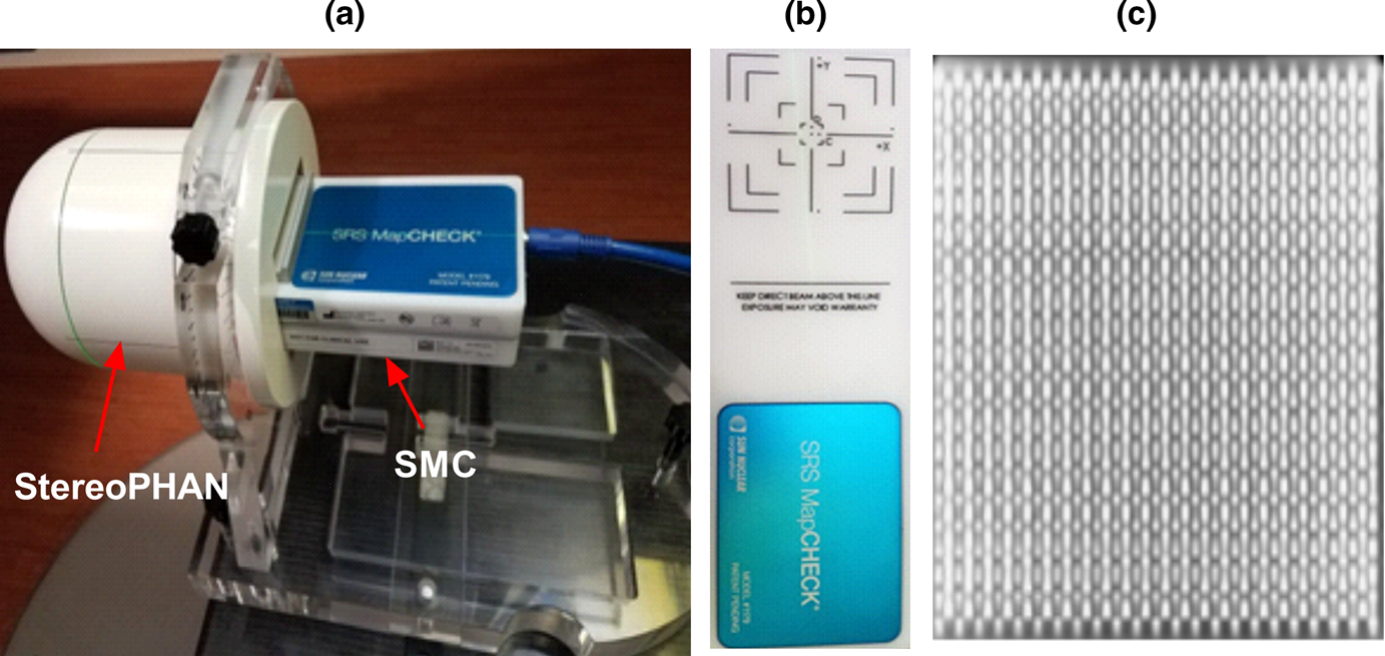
(a) SRS MapCHECK assembled with StereoPHAN, (b) SRS MapCHECK top view, (c) A CT‐based reconstruction of the 7.7 × 7.7 cm^2^ diode array.
